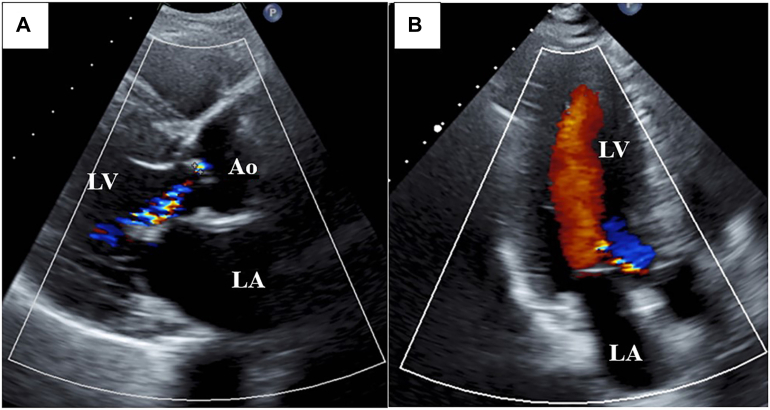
Postoperative transesophageal echocardiography. (a) Long-axis view showing mild residual aortic insufficiency on color Doppler imaging. (b) Continuous-wave Doppler across the aortic valve demonstrating a mean pressure gradient of 8 mm Hg. (Ao, aorta; LA, left atrium; LV, left ventricle.)
